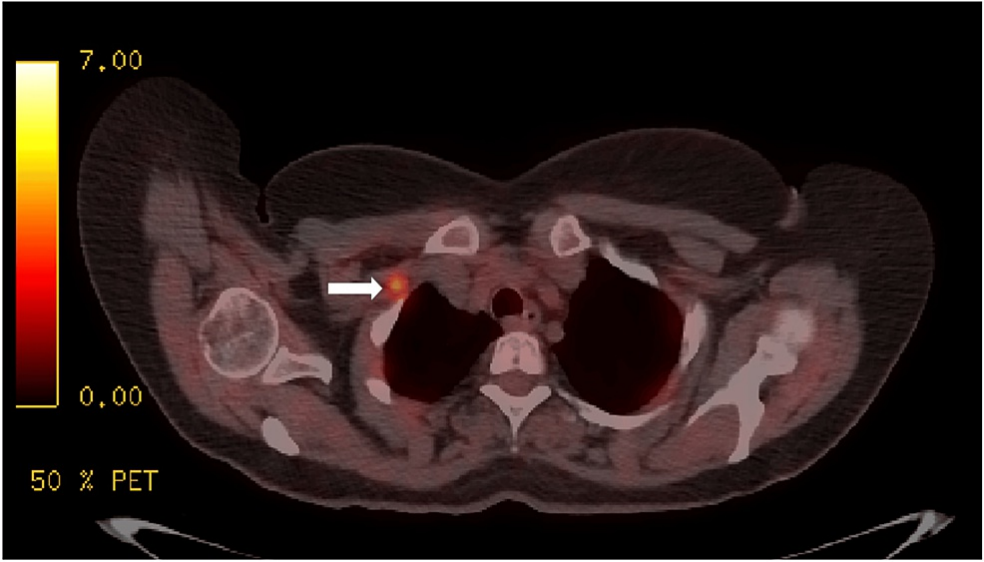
Positron emission tomography/CT showed new lymphadenopathy in the right supraclavicular region, as visually indicated by a white arrow.
